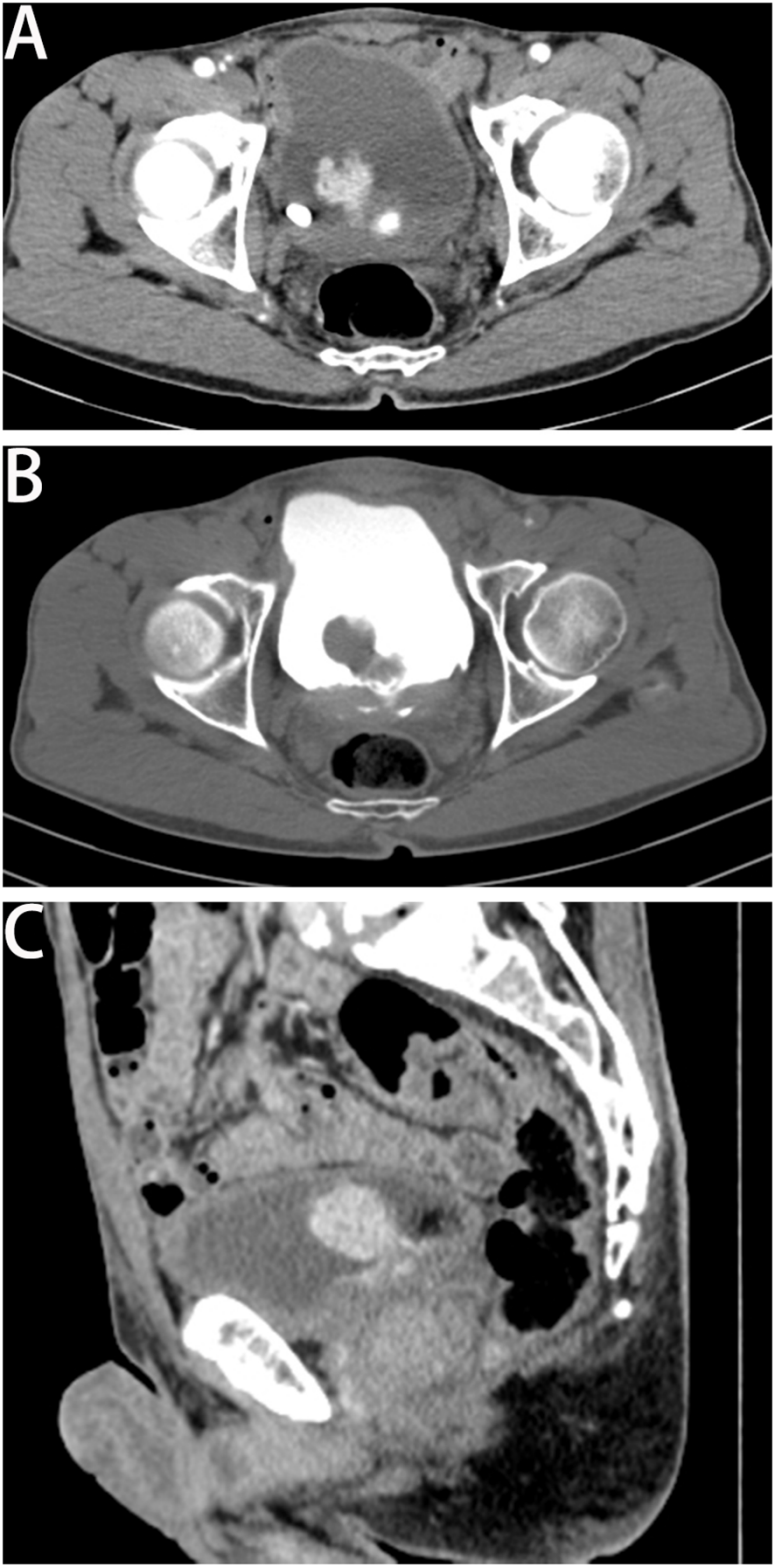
Results of computed tomography urography. Cross sections: panel (A) (arterial phase) and panel (B) (delay 2 phase). Sagittal section: panel (C) showed a lobulated tumor located in the bladder’s posterior wall with its clear boundary. The size was approximately 3.5 × 2.4 × 2.1 cm. The enhanced CT scan indicated that the tumor was significantly enhanced.
